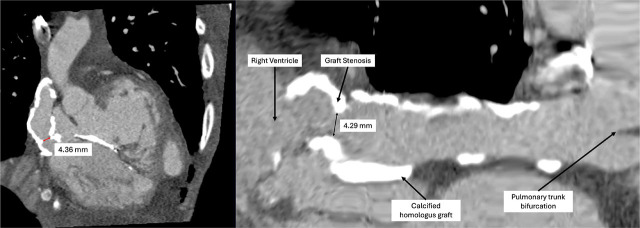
Cardiac CT scan with intravenous contrast: Homograft stenosis-reduction in the diameter of the conduit connecting the right ventricle to the pulmonary artery from 14 mm to 4.29 mm
